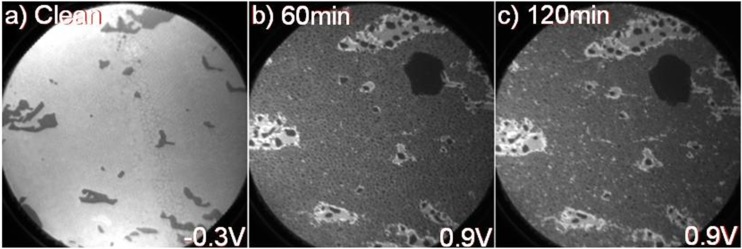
XPEEM image, at a FOV = 25 µm and hv = 150 eV, recorded from (a) the as-grown sample at an electron energy of −0.3 eV; and from the sample after Na deposition and subsequent soft X-ray exposure of (b) 60 and (c) 120 min, respectively, and at an electron energy of 0.9 eV.
